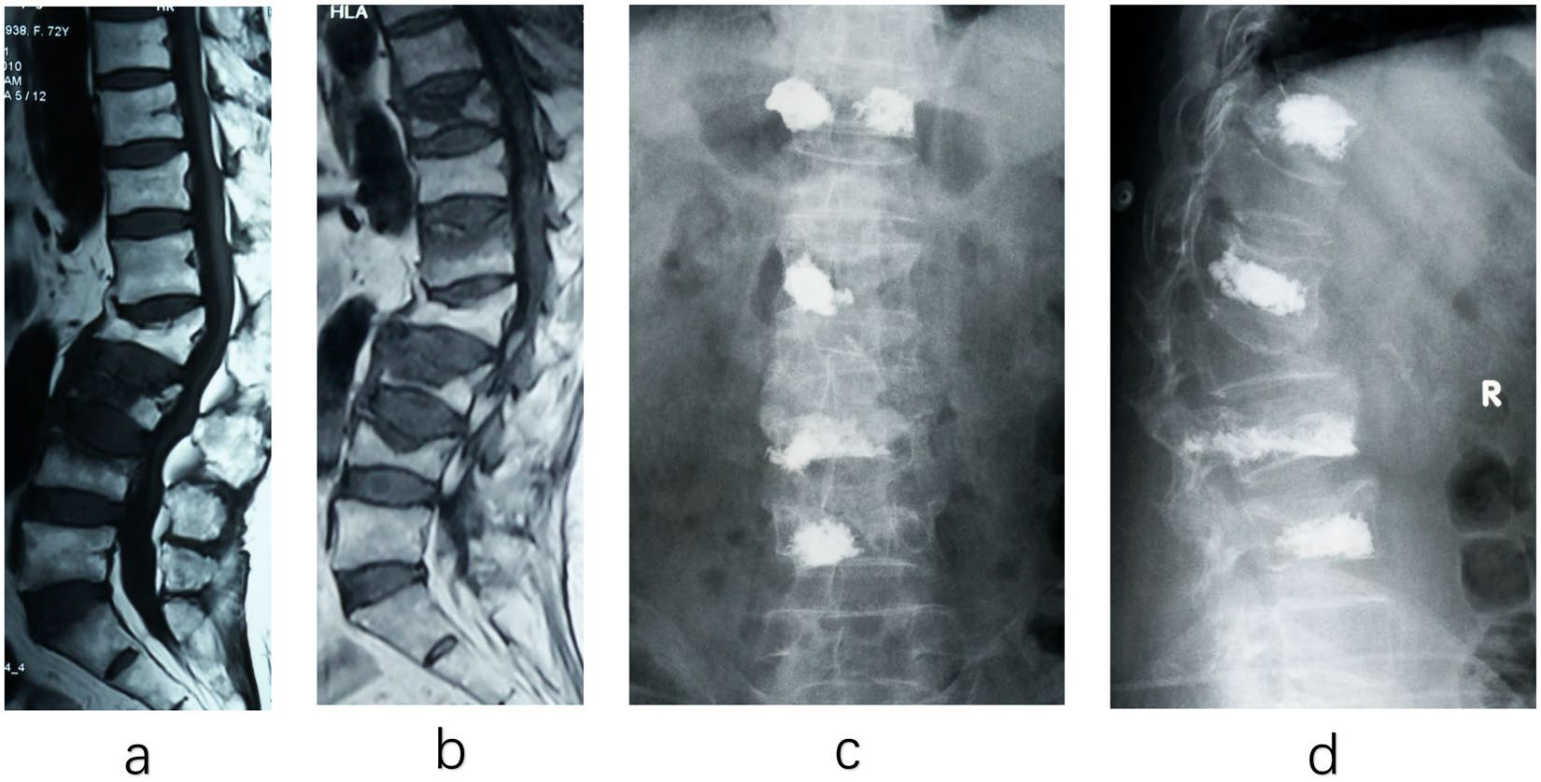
A 70-year-old female patient, thoracolumbar multi-segment osteoporotic fracture, conservative treatment failed with adjacent-segment fractures and then be treated surgically. (a) First admission (2014–06–17): MRI showed lumbar compression fracture in L3, 4 and old lumbar compression fracture in L2. L3 ASTLOF score of 4 points, the total ASTLOF score of 6 points, underwent conservative treatment. (b) The second admission (2014–10–14): MRI showed vertebral collapse in L3 and L4 compared with previous MRI, new thoracolumbar compression fracture in T11 and L1. T11 ASTLOF score of 4 points, the total ASTLOF score of 10 points, underwent PKP. (c,d) Postoperative review of the X-ray showed: bone cement leakage in T11 and L3, no complications were found, and back pain were relieved.
